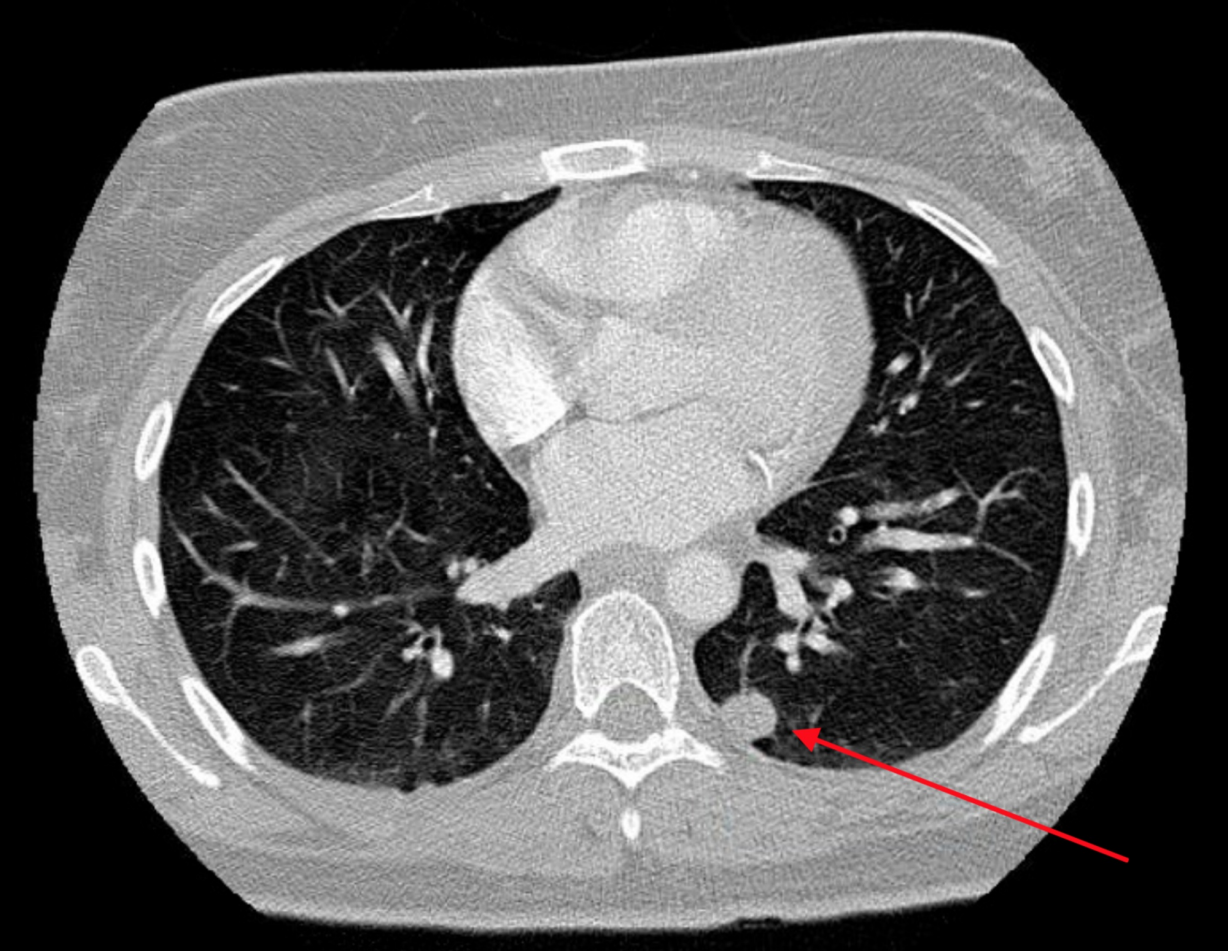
Axial view of computed tomography scan of the chest with contrast Red arrow points to largest metastatic lung nodule located in the left lower lobe and measuring approximately 1.1 cm in the long axis.
